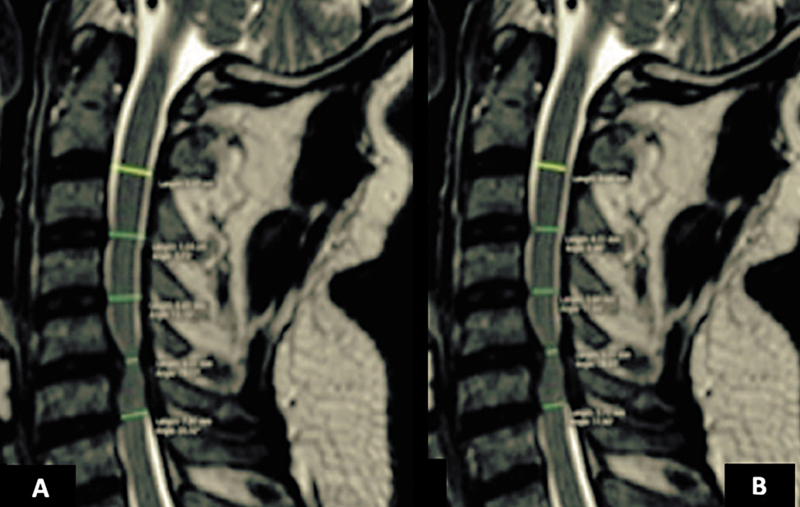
T2-weighted sagittal cervical magnetic resonance imaging scan. (
